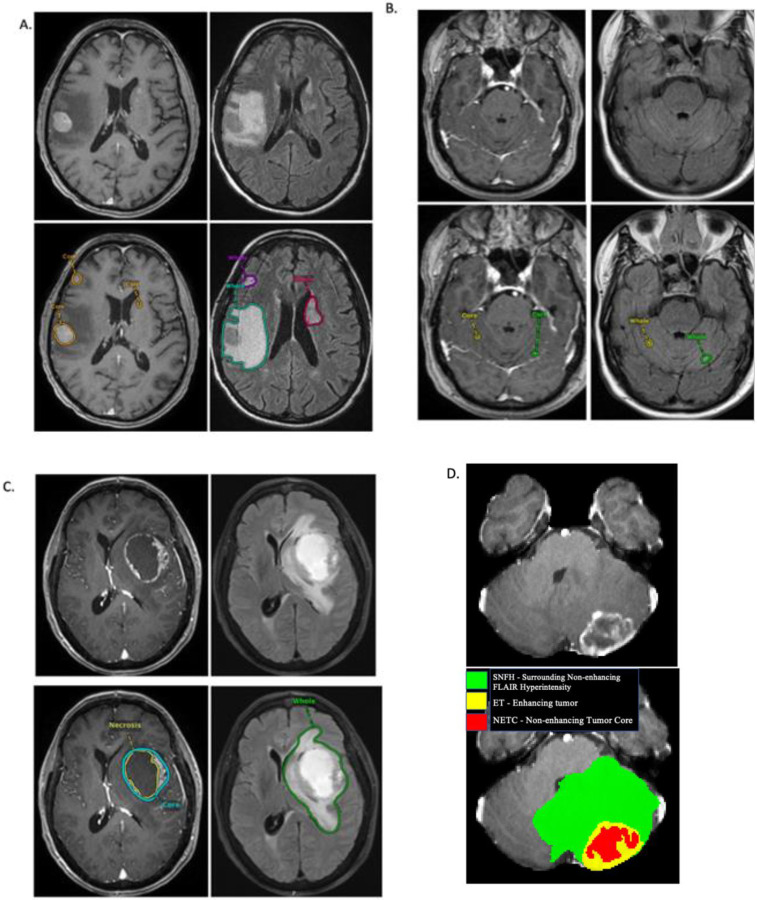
Image panels with the tumor sub-regions annotated in the different mpMRI scans. The image panels A denote the regions considered for the performance evaluation of the participating algorithms and specifically highlight: the enhancing tumor (ET - orange) visible in a T1Gd scan and surrounding edema indicating Surrounding Non-enhancing FLAIR Hyperintensity (SNFH - green, purple, and red). Multiple lesions of various sizes are noted in panel A demonstrating the complexity of annotation of each scan. In panel B, small lesions located within the cerebellar hemispheres are identified and enhancing tumor and surrounding FLAIR hyperintense region are identified on segmentations. In panel C, Visage PACS image of a large centrally necrotic tumor is shown within the left frontal lobe with segmentations of the enhancing tumor (ET), central necrotic portion (SNFH), and peritumoral edema (SNFH). In panel D, ITK-SNAP image of Enhancing tumor (ET) (yellow), Surrounding Non-enhancing FLAIR Hyperintensity (SNFH) (green), and Non-enhancing Tumor Core (NETC) (red) segmentations are demonstrated.
